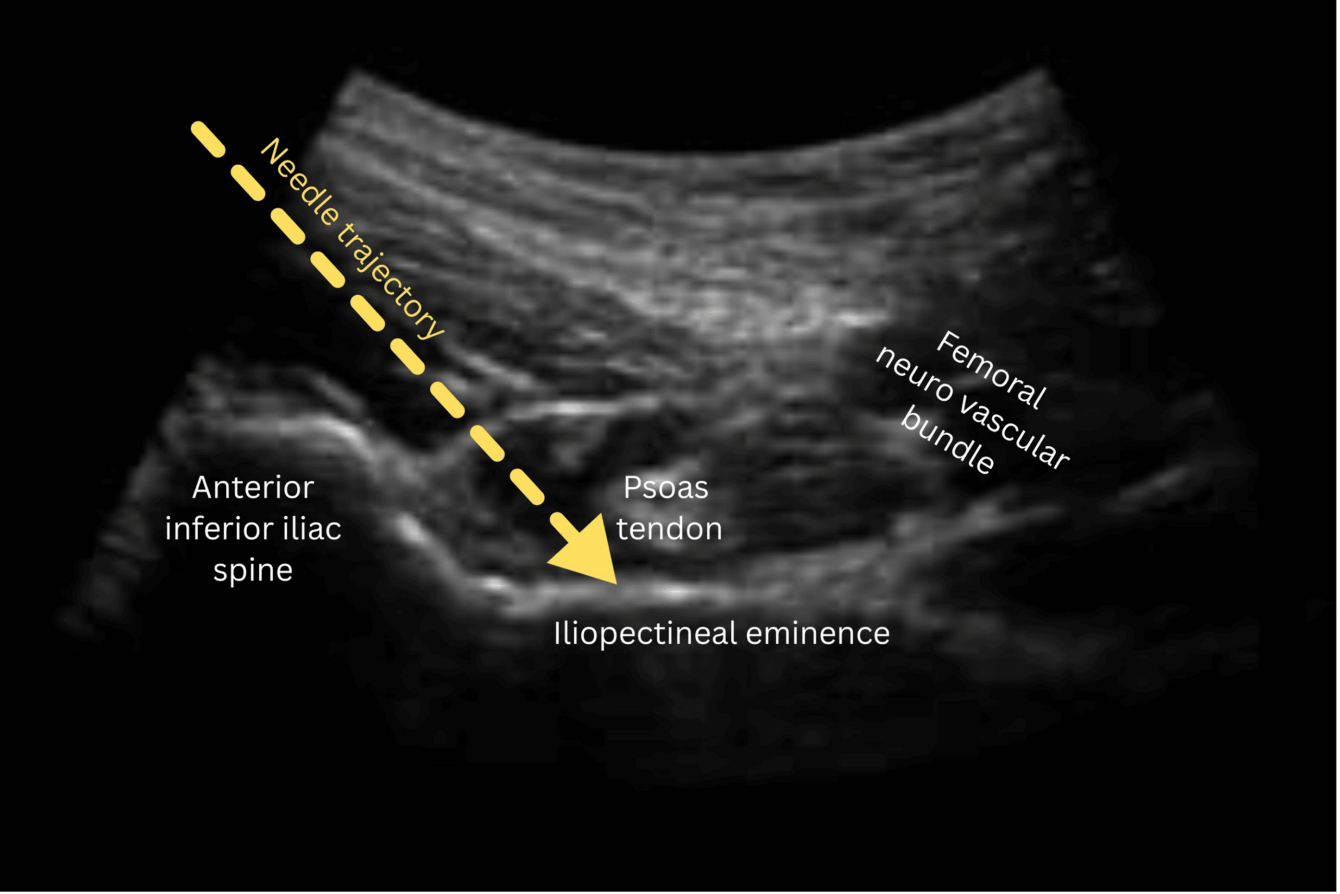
Ultrasound image showing needle trajectory for PENG block targeting the iliopsoas plane between the psoas tendon and iliopectineal eminence. PENG, pericapsular nerve group
